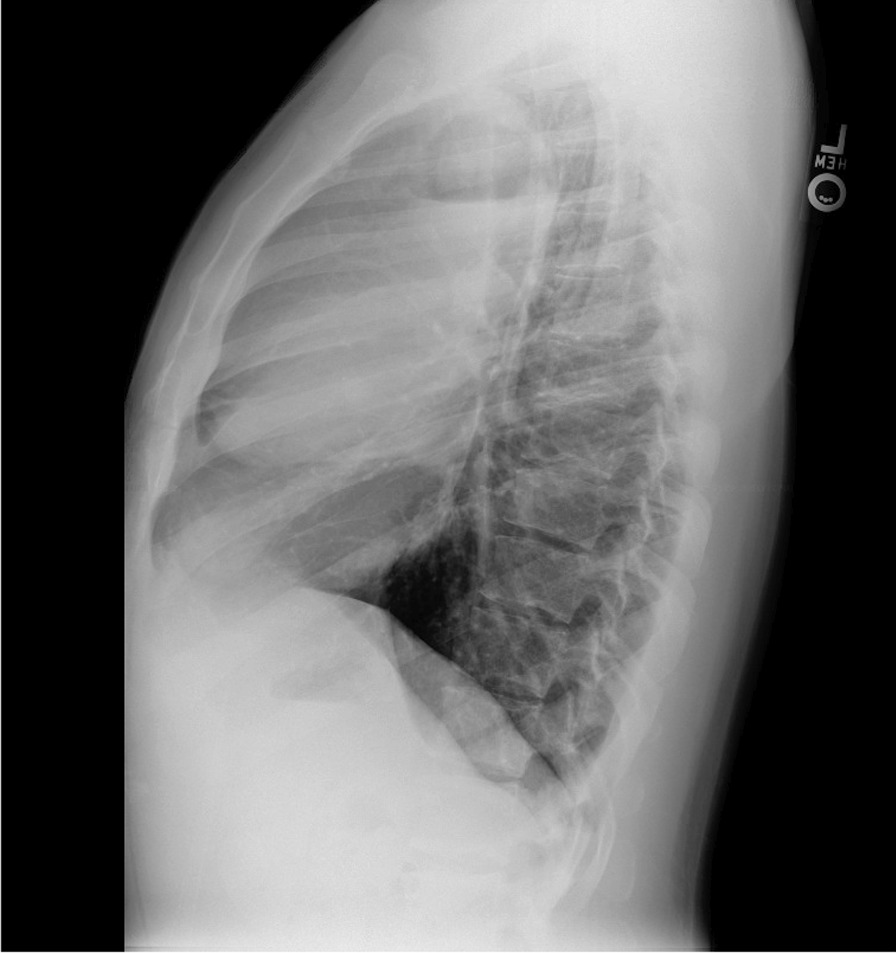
Follow-up chest X-ray (lateral view). Enlarging right upper lobe cavitary lesion
